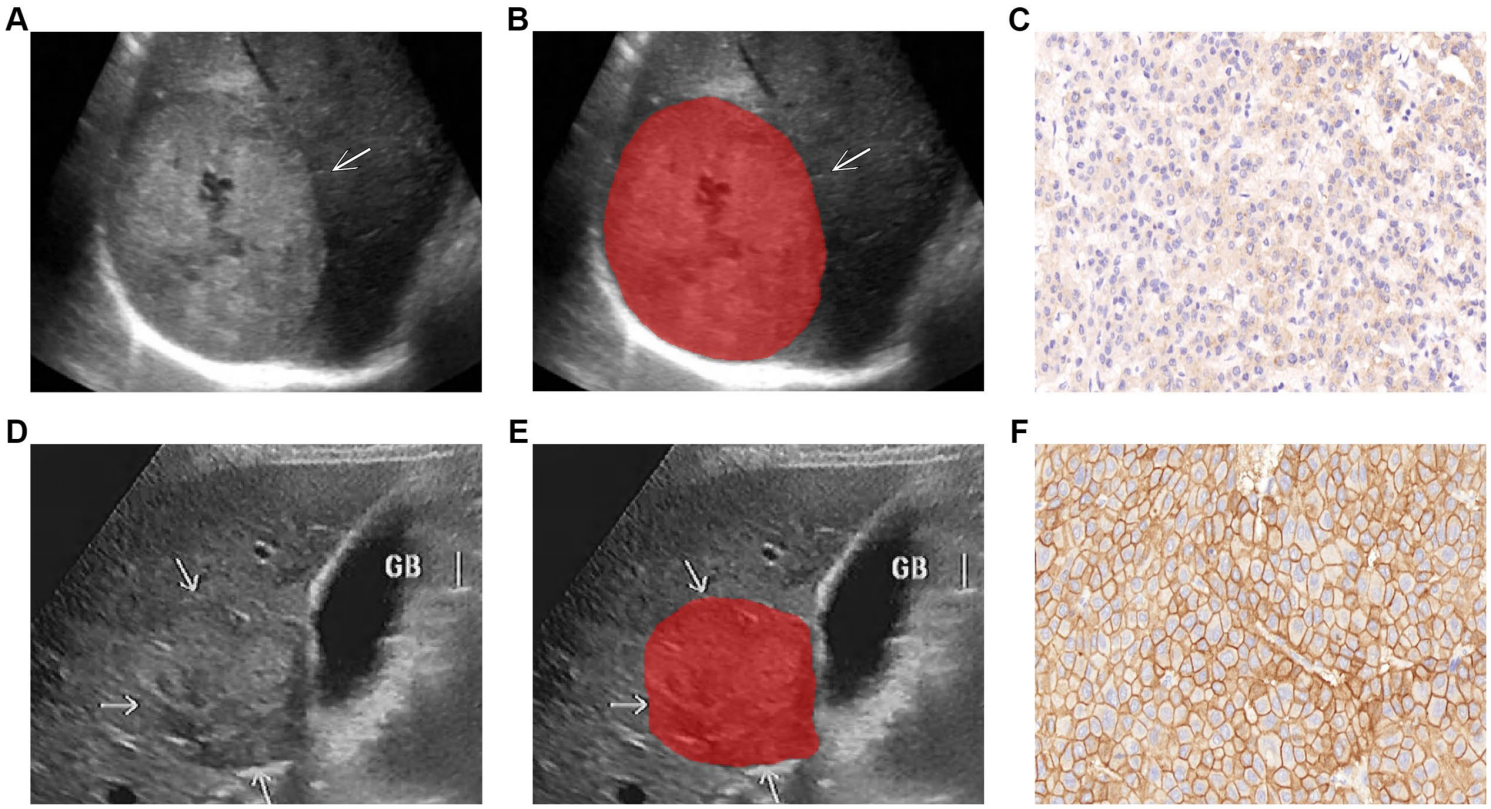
Representative images of lesion segmentation (arrow pointing) and corresponding pathological images of two HCC patients. (A–C) Show the gray-scale ultrasound image, lesion segmentation image, and EGFR^low^ pathological image of a 63-year-old male patient (H<200); (D–F) Show the gray-scale ultrasound image, lesion segmentation image, and EGFR^high^ pathological image of a 55-year-old male patient (H ≥ 200).
